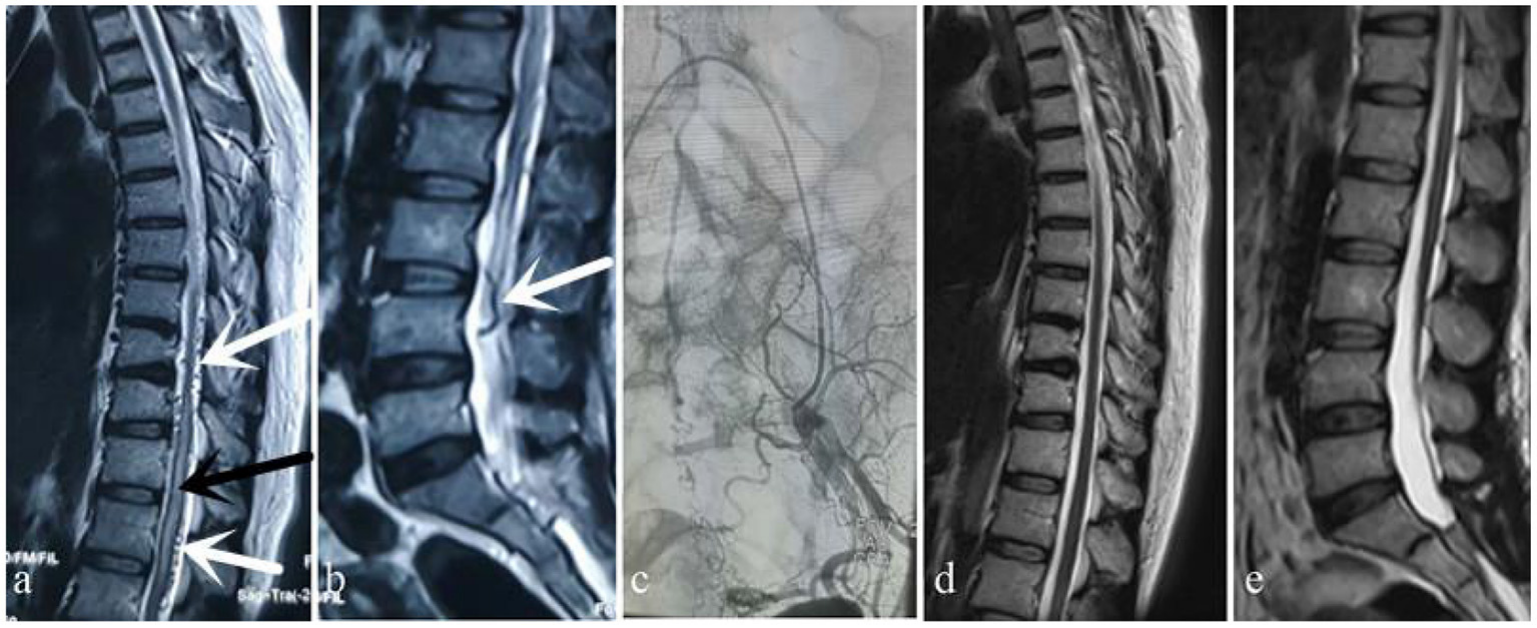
Case 1. A 49-year-old woman sought treatment 7 months after the onset of initial clinical symptoms of numbness and weakness of the bilateral lower limbs with urinary disturbance. The patient was first evaluated at the orthopedics clinic. An orthopedic surgeon suspected a spinal DAVF based on thoracic and lumbar MRI, which showed thoracic spinal cord edema and beaded changes on the dorsal side (a) (white arrow: “beaded” dilated flow void signals, black arrow: spinal cord edema) and tortuosity and dilation of the lumbar dural surface vessels (b) (white arrow: tortuous flow void signals). After MDT consultation, angiography performed by a neurosurgeon showed that the fistula was located in the sacrococcygeal region (c). MRI 6 months after surgery showed that spinal cord edema and signs of flow voids had subsided (d,e).
